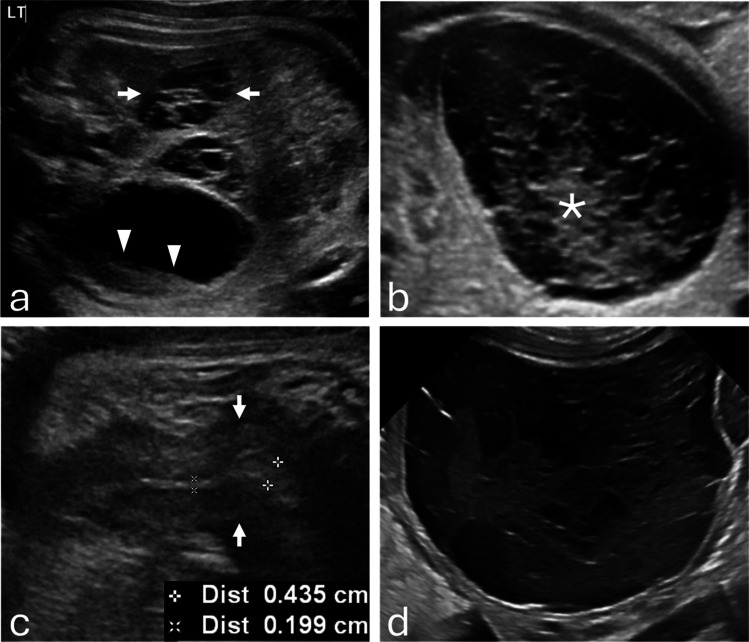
a Prenatal grayscale ultrasound at 30 weeks demonstrates a hormonally stimulated right ovary containing a hemorrhagic cyst with a fluid–debris level (arrowheads) and preserved stroma with follicles. The left ovary is also hormonally stimulated with multiple follicles (arrows). b At 36 weeks, the cyst evolved to a reticular pattern with echogenic, non-shadowing retractile clot (asterisk). c Sagittal grayscale ultrasound shows the fetal uterus (arrows) with a thickened endometrium at the fundus measuring > 2 mm. d Postnatal grayscale ultrasound at day 4 demonstrates a persistent hemorrhagic ovarian cyst measuring 74 mL in volume. The infant underwent surgery, which confirmed a necrotic ovarian cyst with hemorrhage and calcifications due to prenatal torsion
